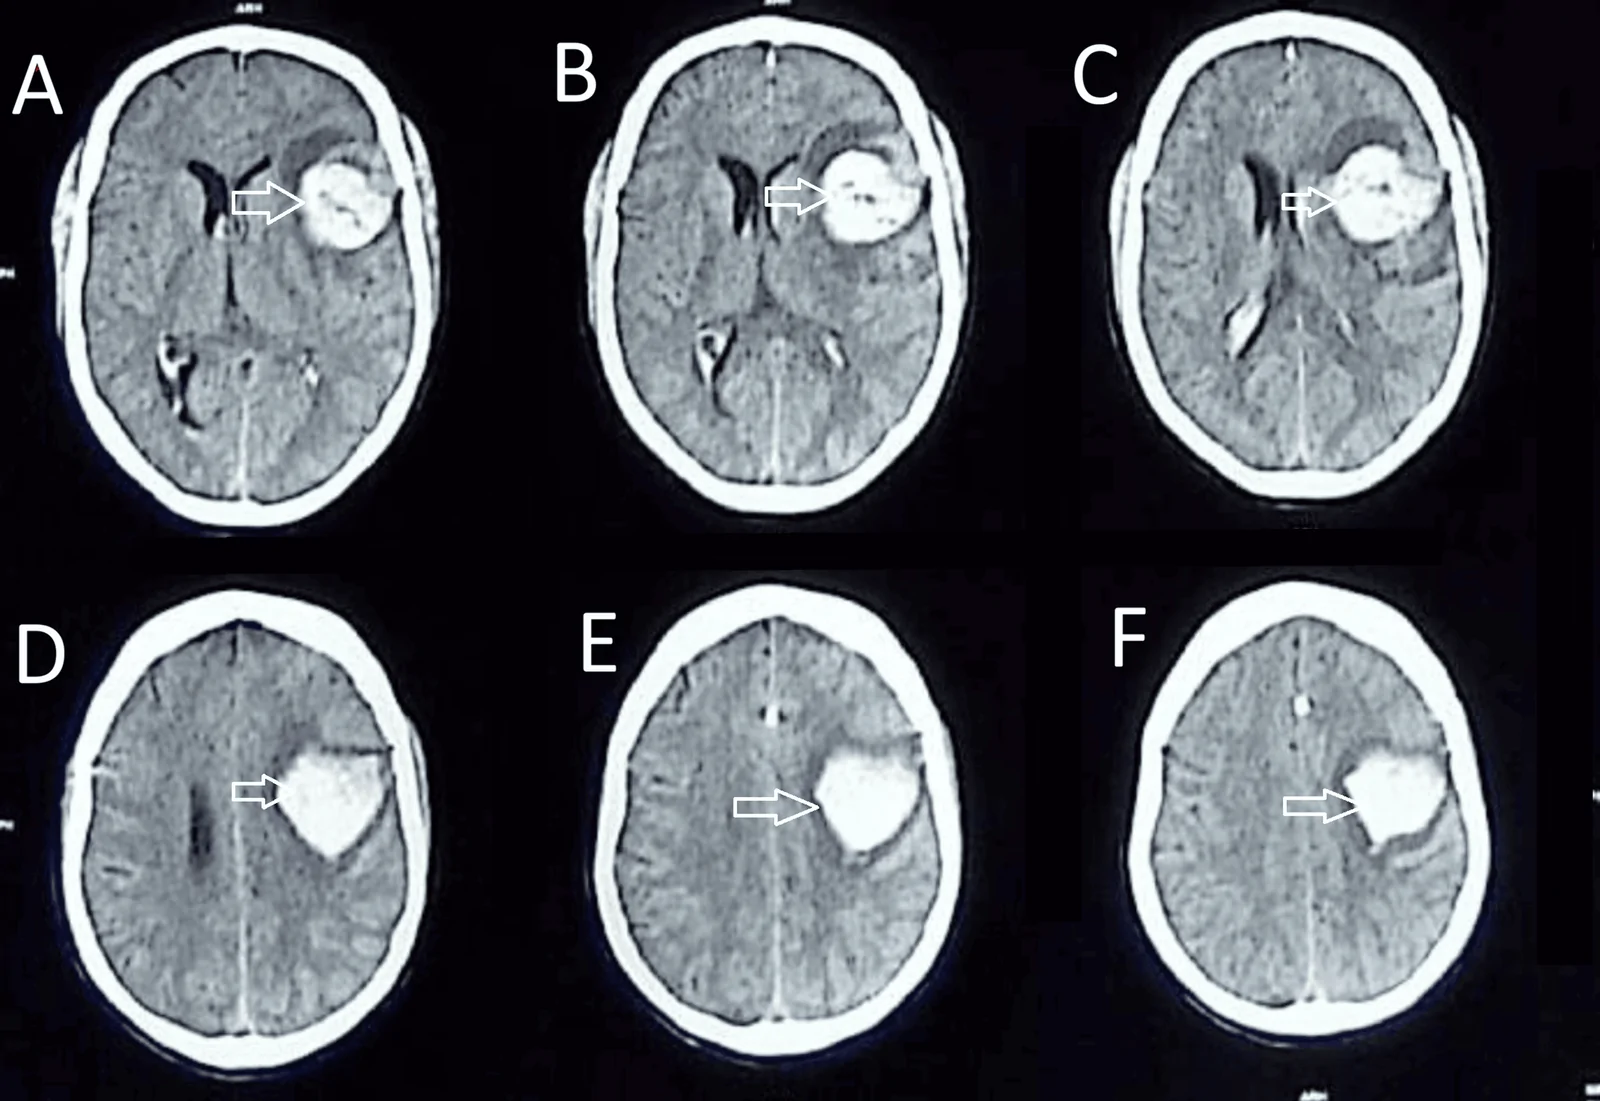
Initial non-contrast CT brain Sequential axial images (A-F) demonstrate a large left fronto-temporo-parietal lobar hemorrhage (white arrows) with surrounding edema.
